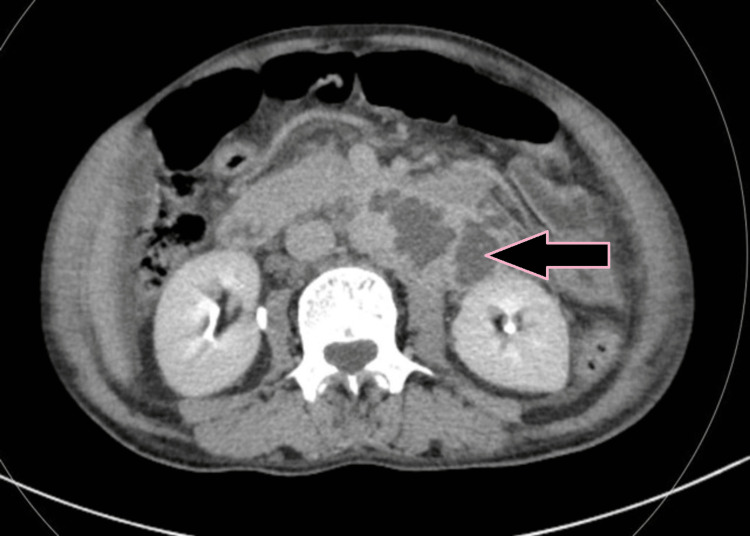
DECT shows lymph nodes with characteristic low attention center and peripheral rim (case 3). DECT: dual-energy computed tomography
